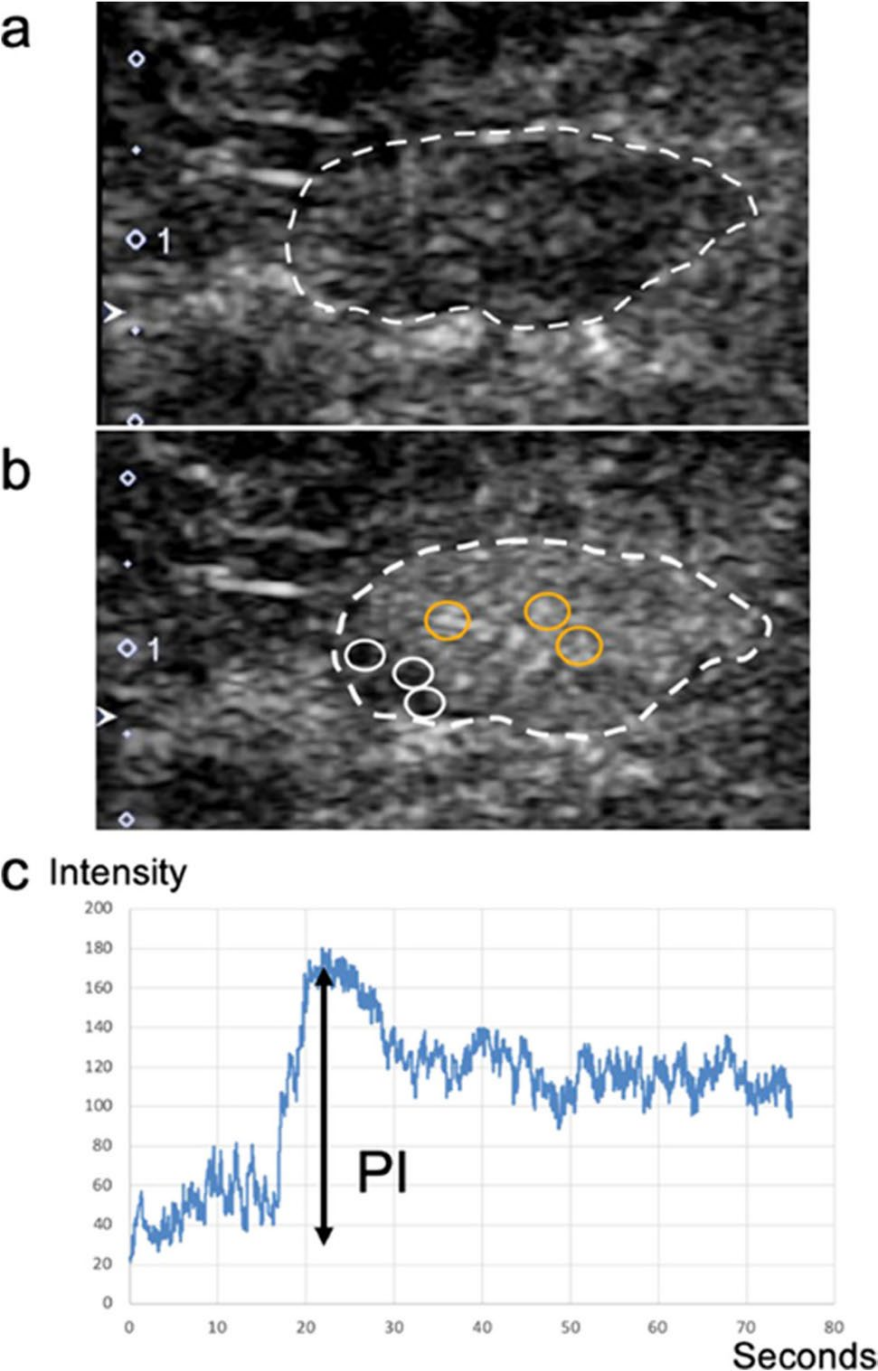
Methods for placing regions of interest (ROIs) in sentinel lymph nodes (LNs) and acquiring time-intensity curves (TICs) for intraoperative perfusion contrast-enhanced ultrasound (CE-US) in uterine cancer. a Intraoperative pre-contrast US revealing manual segmentation covering the entire LN volume (dotted line). b Intraoperative perfusion CE-US at the arterial phase. Inside the LN (dotted line), three ROIs (orange) were selectively placed where the strongest enhancement was observed. The other three ROIs (white) were selectively placed where the weakest enhancement was observed. c TICs were generated from the ROIs with time on the x-axis and signal intensity on the y-axis. Peak intensity (PI) was measured from the TIC. The maximum PI value from the three ROIs with the strongest enhancement was recorded as PImax. The minimum PI value from the three ROIs with the weakest enhancement was recorded as PImin. Reprinted from Ref. 65 with permission
